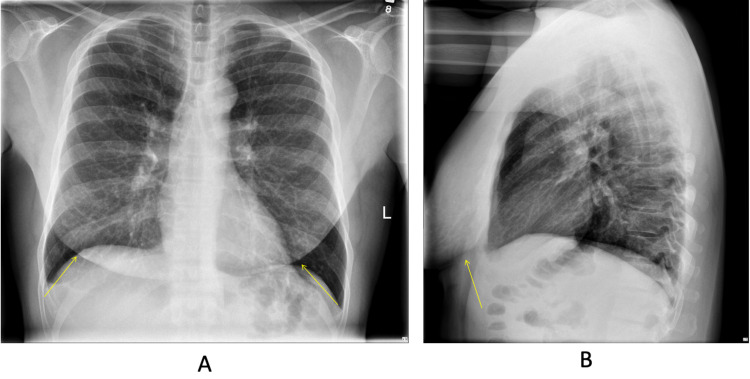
Frontal (A) and lateral (B) chest radiographs show bilateral symmetric soft tissue densities.
